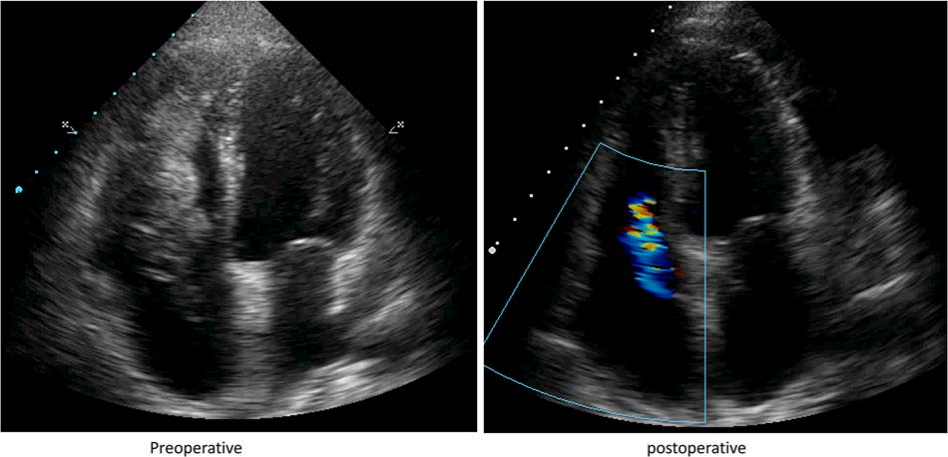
Transthoracic echocardiography revealed the presence of an extremely mobile, lobulated cardiac mass protruding from the right atrium through the tricuspid valve into the right ventricle (image left). The image on the right side shows a postoperative view, highlighting the changes found after the surgical intervention.
